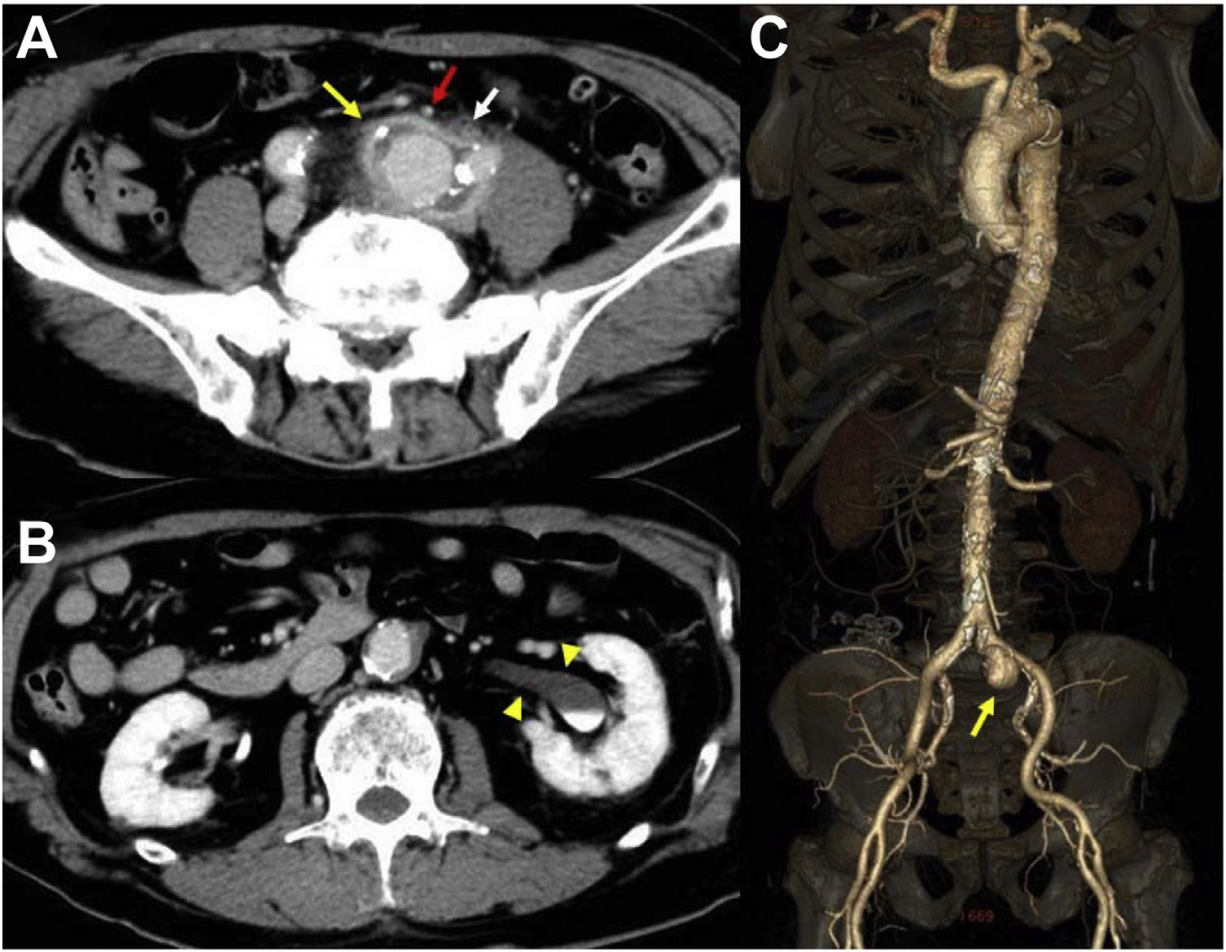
Enhanced abdominal computed tomography (CT) (A, B) and multidetector computed tomographic angiography (C) findings showed a 27 × 32 mm sized saccular pseudoaneurysm (yellow arrows) with the surrounding inflammation, involving inferior mesenteric artery (red arrow) and left ureter (white arrow), in the left common iliac artery (A, C). The compression of the left ureter led to left hydronephrosis (yellow arrowheads) (B). (For interpretation of the references to colour in this figure legend, the reader is referred to the web version of this article).
